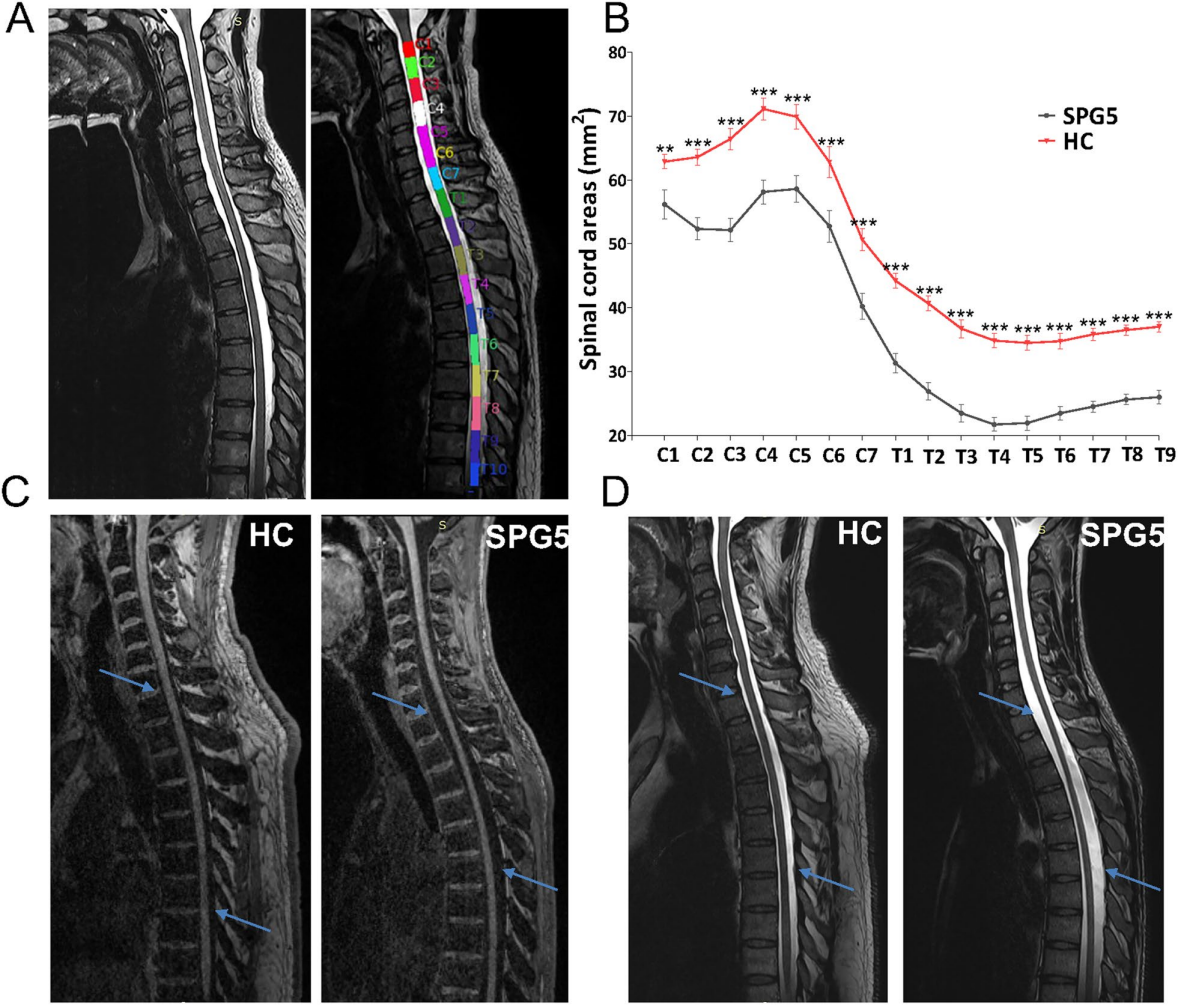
Quantitative MRI to assess spinal cord area. A Processing included 3 steps: (1) composing the cervical and thoracic spinal cord with 3D-T2W-SPACE; (2) segmentation and labeling the spinal cord; (3) measurement of spinal cord cross-sectional area (SCA) and sectional diameter (RL and AP). B Area of spinal cords (C2-T9) in SPG5 patients and matched healthy controls, comparisons performed using Mann–Whitney test, *P* < 0.01, P* < 0.001. C, D Representative MRIs of SPG5 and healthy control. In SPG5 patients, a significant thinning in the spinal cord was observed (blue arrow) in the 3D T1-weighted and 3D T2-weighted sequence, respectively
